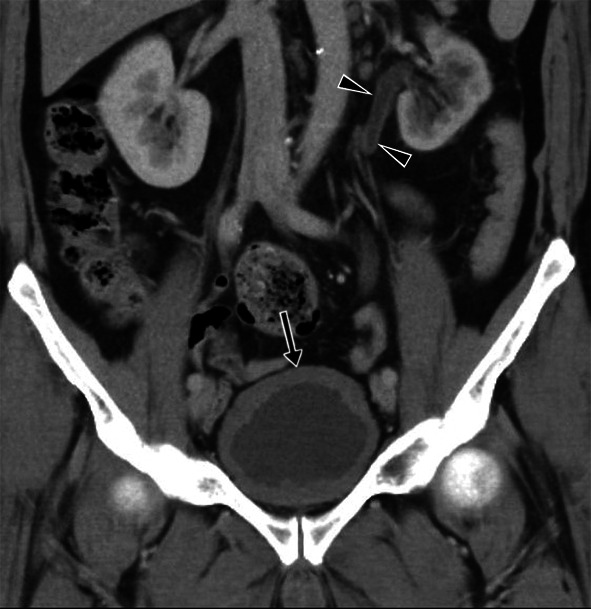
A 47-year-old male. Coronal post-contrast CT scan shows diffuse thickening of the bladder wall (arrow) and dilated left proximal ureter (arrowheads). Urinary TB
